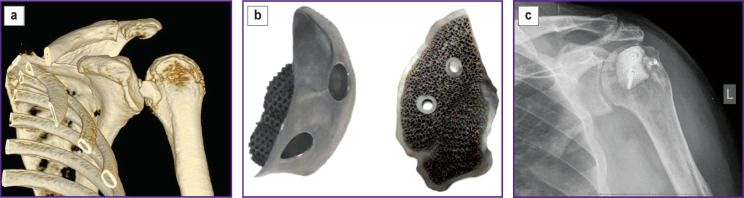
Replacement of the humerus head defect with a customized implant: (a) a CT scan of the right shoulder joint before surgery; (b) a 3D-printed customized implant; (c) an X-ray image of the left shoulder joint after surgery
